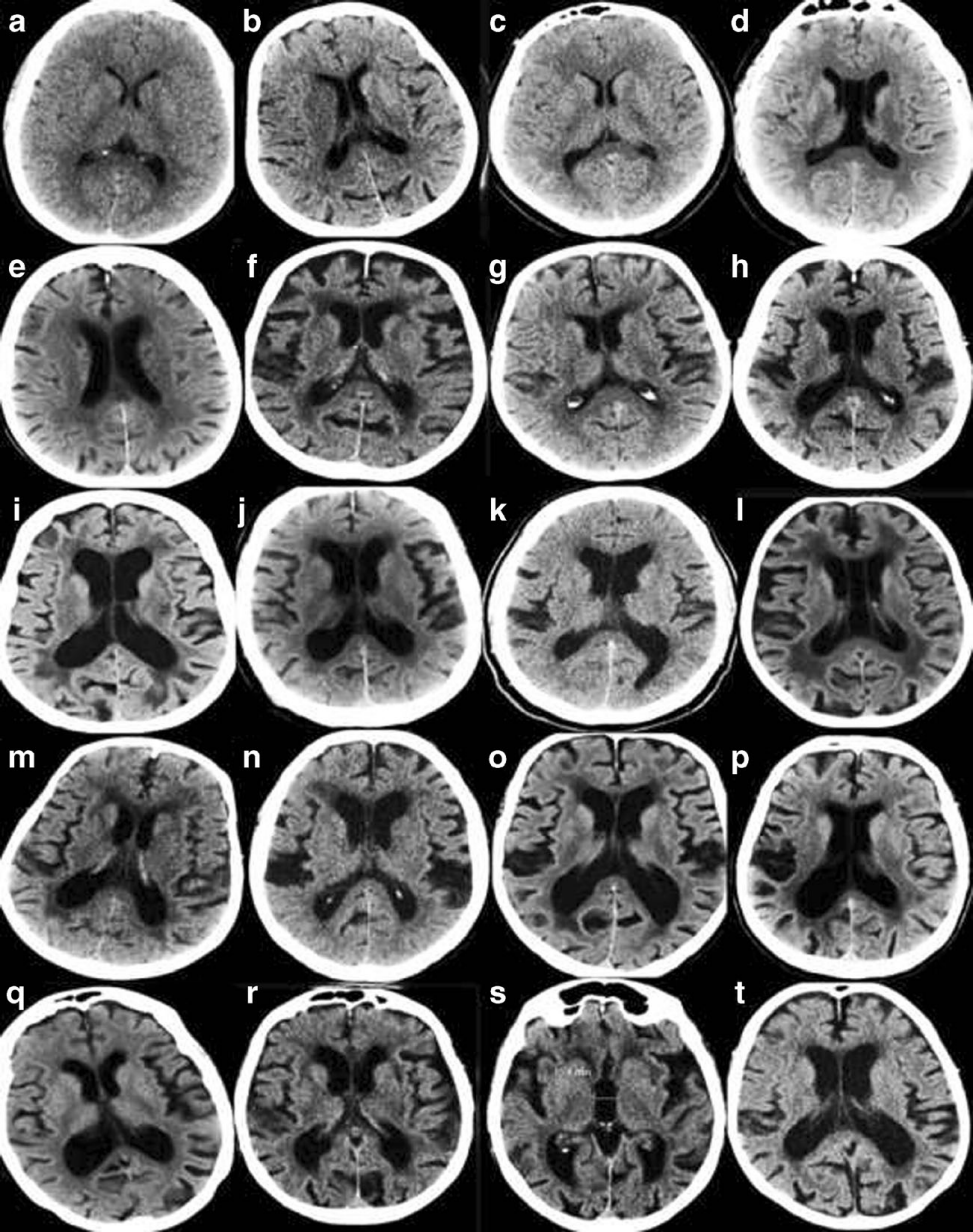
A. Widening of the ventricles on MRI. Interpretation key. Numbers equal the corresponding image in the supplementary PDF file. Appropriate wording for widening of the ventricles is: no, mild, moderate or severe widening. a/1) There are no visual rating scales for the size of the ventricles. Compare to the widening of a 25-year-old healthy individual. b/2) A third ventricle with a diameter of >10 mm is pathological at any age. c/6) No widening. d/16) No widening. e/17) In between no widening and mild widening. The third ventricle is wider than usual. f/20) Mild widening. Note the slightly wide frontal horn on the left side due to the frontal infarction. g/7) Mild widening h/9) Mild widening. i/15) Approaching mild widening. j/12) Mild bifrontal widening and no widening of posterior horns. k/11) Mild bifrontal widening and a left posterior horn with moderate widening. l/3) In this case: mild, with a moderate widening of the posterior horns. m/5) Mild widening of the frontal horns and moderate of posterior horns. n/8) Mild widening, with a moderate widening of frontal horns. o/19) In between mild and moderate widening but closer to moderate. p/13) Mild central widening, with the right posterior horn a bit wider, but not yet moderate. q/10) Mild widening of frontal horns and moderate of the posterior horns, especially the left posterior horn. r/14) Moderate widening, especially of posterior horns. s/18) Severe dilatation of third ventricle and moderate widening of the posterior horns. t/4) Severe widening of the ventricles, especially of the frontal horns. B. Widening of the ventricles on CT. Interpretation key. Numbers equal the corresponding image in the supplementary PDF file. a/1) No widening. The normative key. b/7) No widening, right deep MCA infarction is seen in the image. c/3) No widening d/19) No widening but wide cavum septi pellucidi et vergae. e/11) Nearly no widening. f/14) Between no widening and mild. g/6) Normal to mild widening. h/9) Mild widening. i/12) Mild widening. j/13) Mild widening. k/18) Mild widening of ventricles. l/17) Mild widening of lateral ventricles. Cavum septi pellucidi et verge makes ventricles look wider. m/15) Mild widening of the posterior horns without exceeding into moderate widening. n/20) Mild widening of frontal horns and a mild to moderate widening of the posterior horns. o/10) Mild widening with a moderate widening of the left posterior horn. p/8) Mild widening with a moderate widening of the right posterior horn. q/16) Mild widening of frontal horns and moderate widening of posterior horns. r/4) Mild to moderate widening s/5) A pronounced widening of the third ventricle. Maybe this indicates atrophy of the basal ganglia and thalami. t/2) Moderate widening
